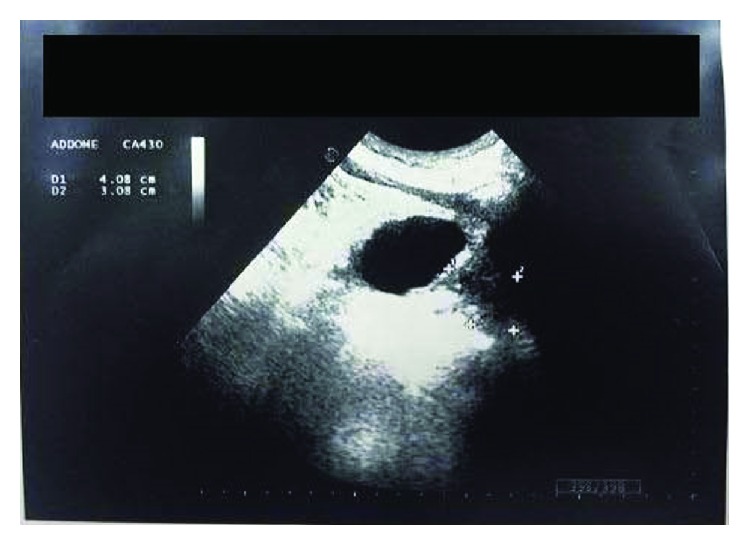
Ultrasound aspects of the renal hematoma.
